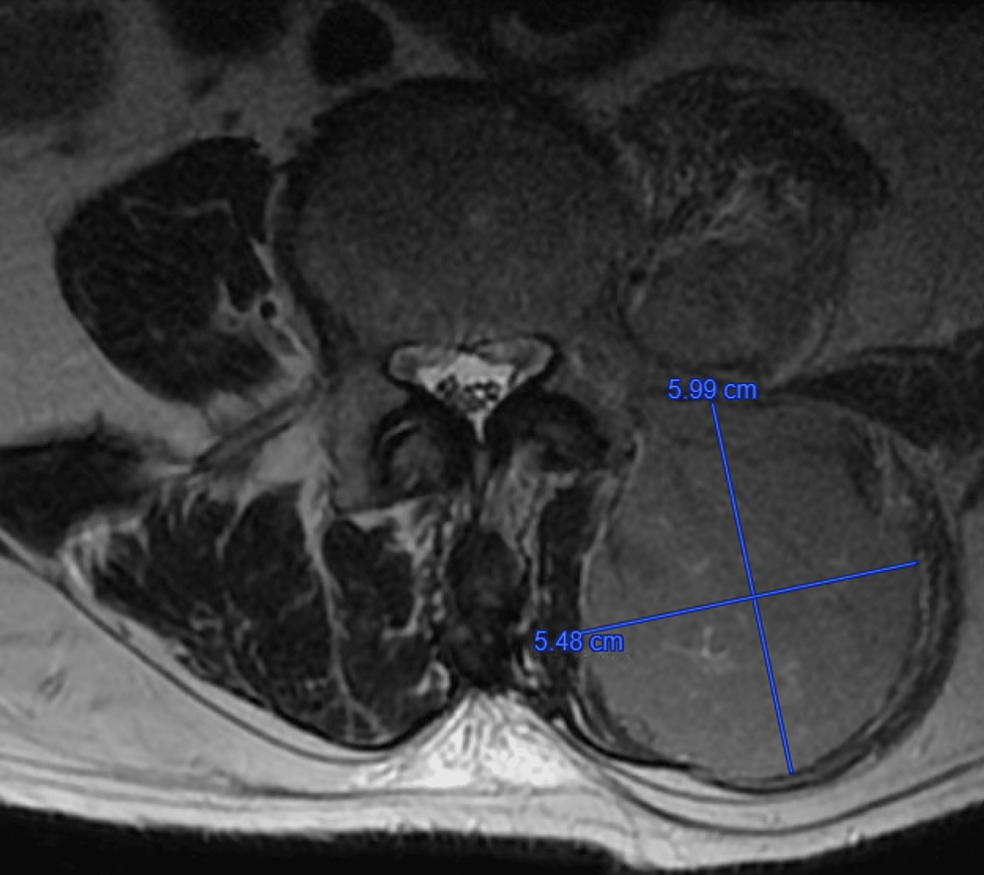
The larger constituent situated within the left posterior paraspinal muscle measuring 5.48 x 5.99 cm in T2-weighted axial plane view
